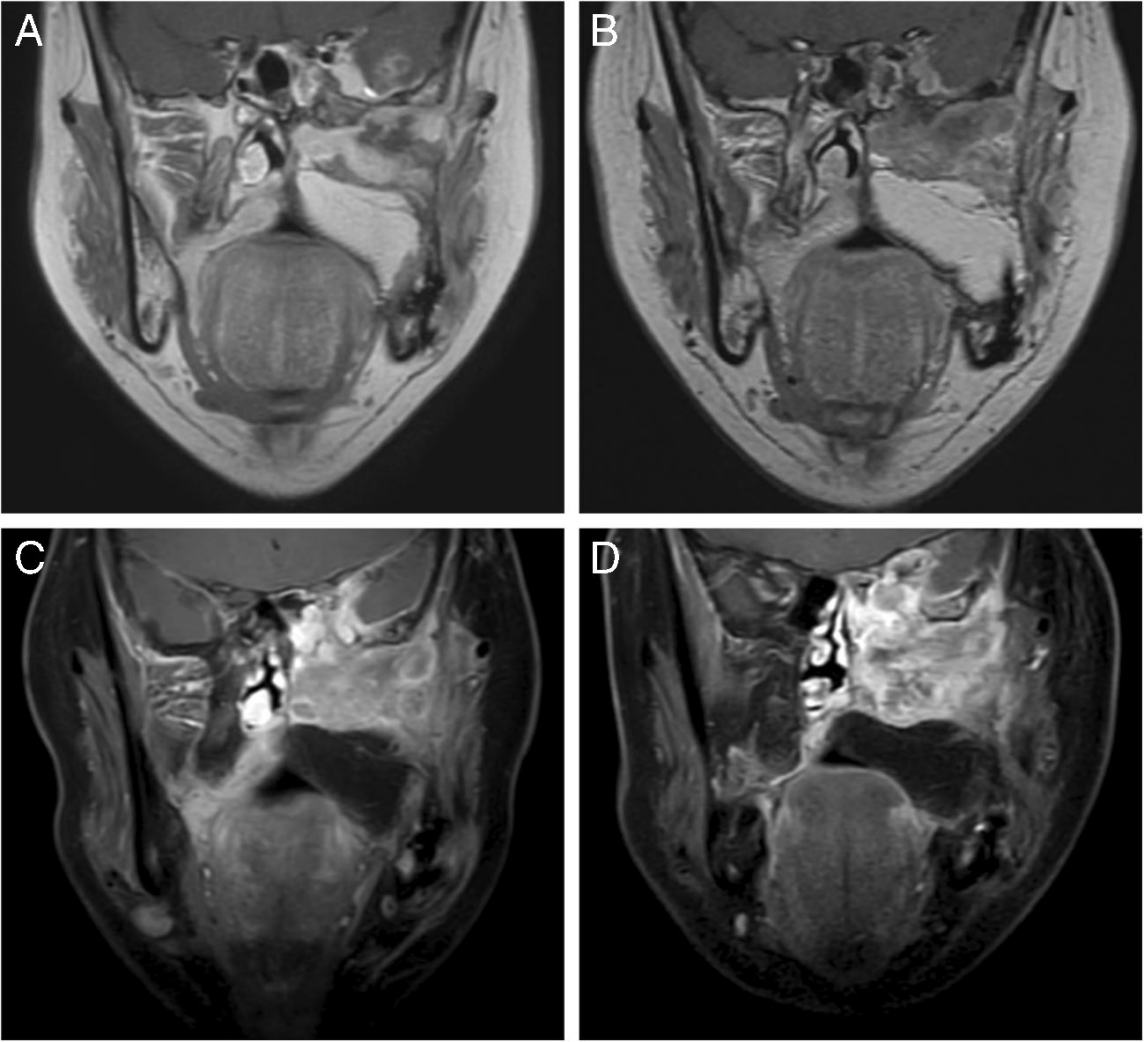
Magnetic resonance imaging (MRI) demonstrated recurrent ACC involving the skull base and intracranial invasion before surufatinib treatment (A, July 22, 2021), on the second month of surufatinib treatment (B, September 23, 2021; the tumour size was reduced), on the fourth month of surufatinib treatment (C, November 17, 2021; the tumour size was not further reduced), and before admission (D, December 21, 2021; the tumour size was evidently larger)
